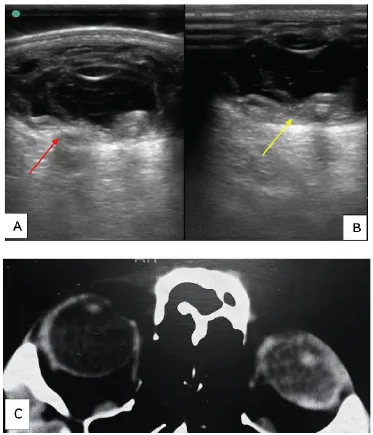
Case 4. A patient with suspected vitreous haemorrhage and globe rupture: A) using modified water bath technique (red arrow); B) using conventional method on the same eye using copious amount of gel (yellow arrow); and C) computed tomography suggestive of left globe injury.
